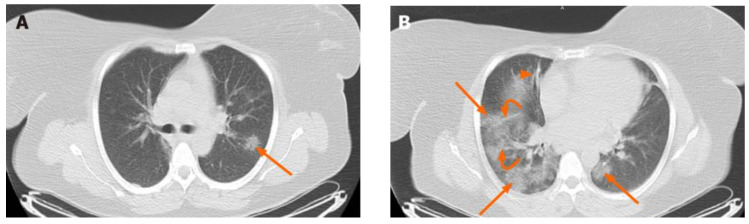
Chest radiographsof a 46-year-old female patient with fever and dry cough [9]. Axial chest computed tomography shows bilateral multifocal ground glass opacities (arrows; (A,B)), peribronchial interstitial thickening (arrowhead; (B)) and reticular opacities (curved arrows; (B)), consistent with coronavirus disease 2019 pneumonia.
